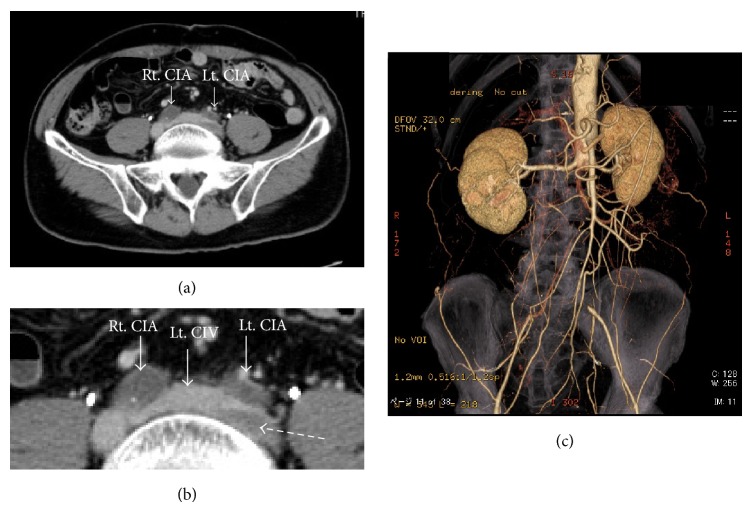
(a) The left common iliac vein (Lt. CIV) was compressed by the left common iliac artery (Lt. CIA) resulting in formation of a venous thrombus at this point. (b) The thrombus was observed in left common iliac vein (dotted arrow). (c) CT showed that the terminal aorta was occluded from the level of renal artery to the bilateral common iliac arteries.
